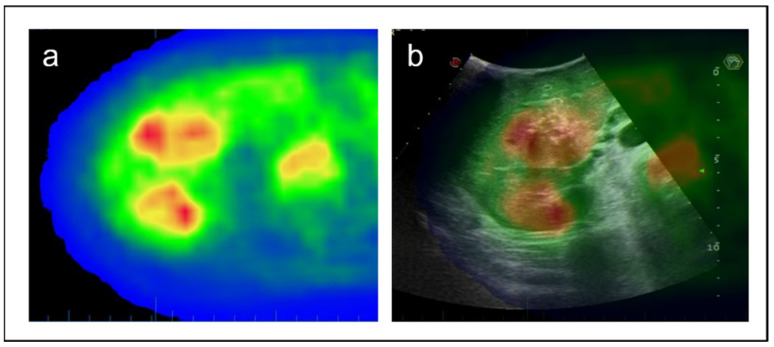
Navigation technology with the intraoperative fusion of preoperative PET-CT and IOUS. The tumour areas having different uptake at PET-CT are identified in vivo during surgery. (a) PET axial view of ICC; (b) Intraoperative fusion of PET-CT and IOUS images.
